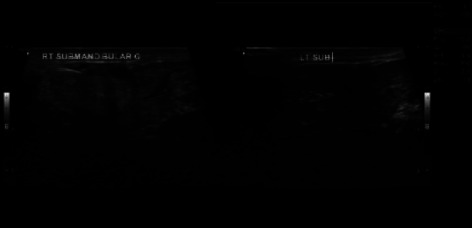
Neck ultrasound of both submandibular glands which shows left submandibular gland coarse echotexture, normal size, and vascularity.
